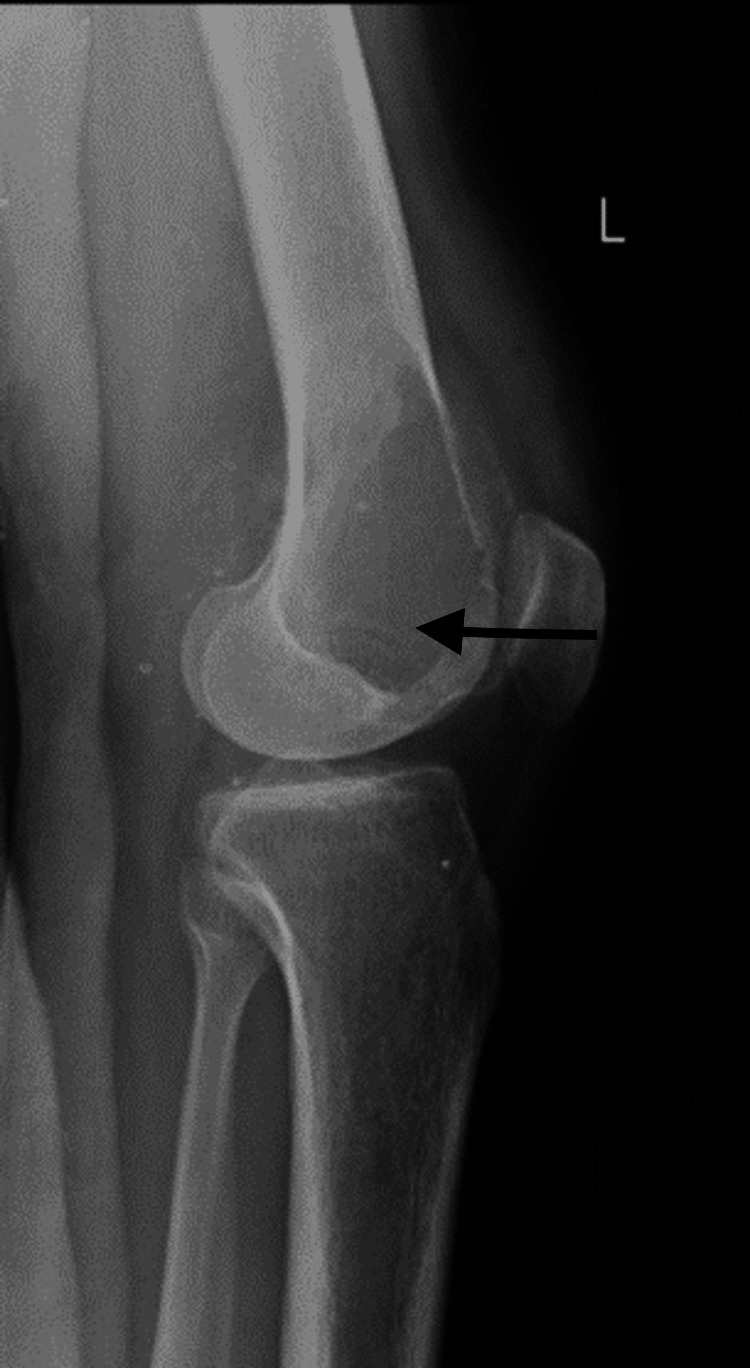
Plain radiograph of the knee, lateral view shows lytic lesion over the lateral femoral condyle
